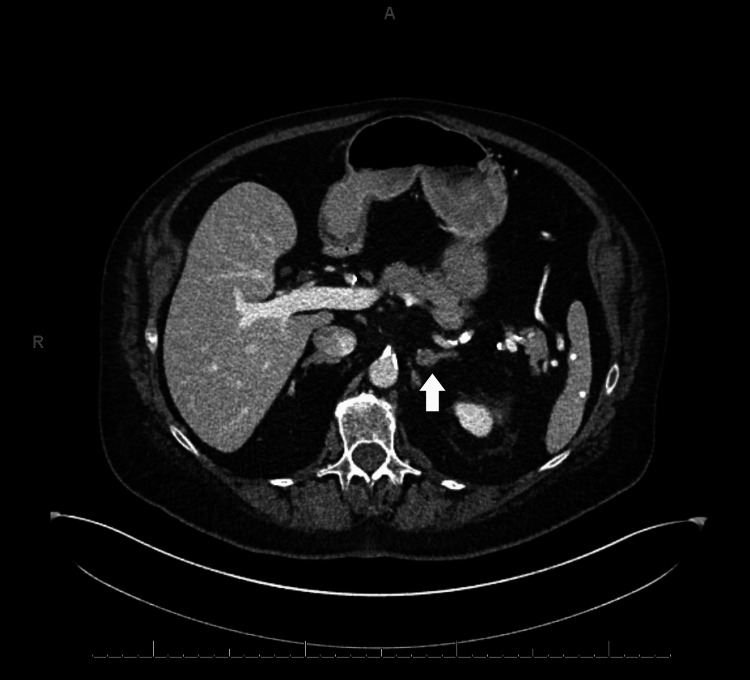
CT scan with contrast of the abdomen showed a 12 mm left adrenal nodule CT: computed tomography
